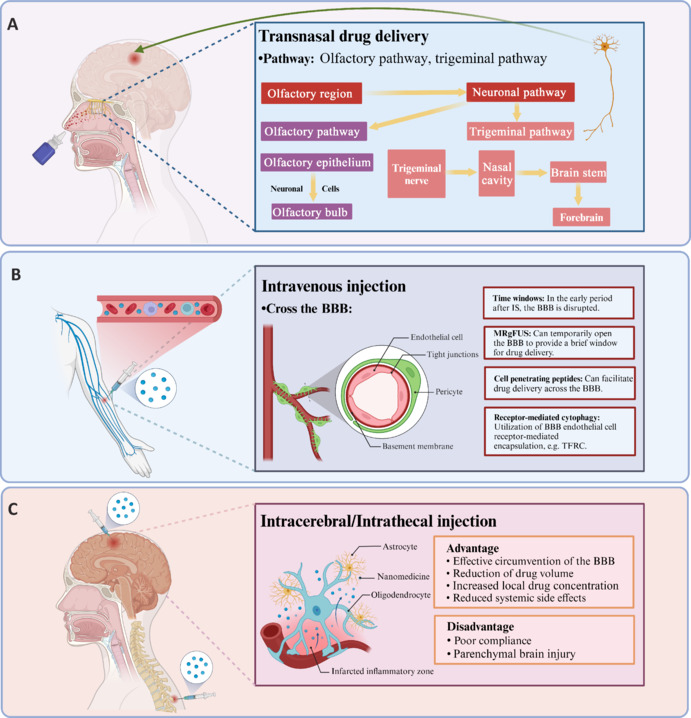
Delivery methodologies of nanomedicine. (A) Two pathways for transnasal medication administration. (B) Intravenous medication administration requires traversing the BBB. (C) Intracerebral injection and intrathecal injection. Created in BioRender. Erter, E. (2025). https://BioRender.com/r87y104. BBB: Blood–brain barrier; MRgFUS: magnetic resonance-guided focused ultrasound; TFRC: transferrin receptor.
